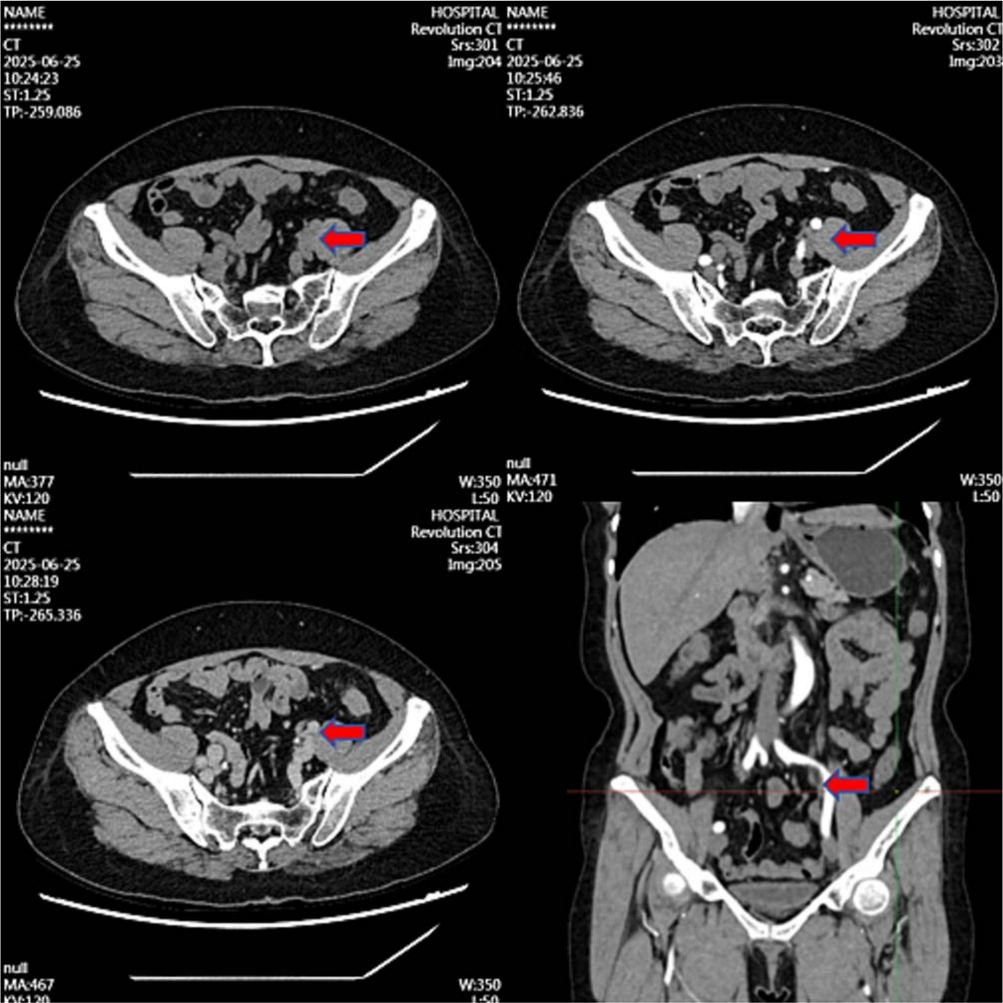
Contrast-enhanced CT image at 36-month follow-up demonstrating near-complete resolution of previously described left iliac vessel mass following medical therapy.
